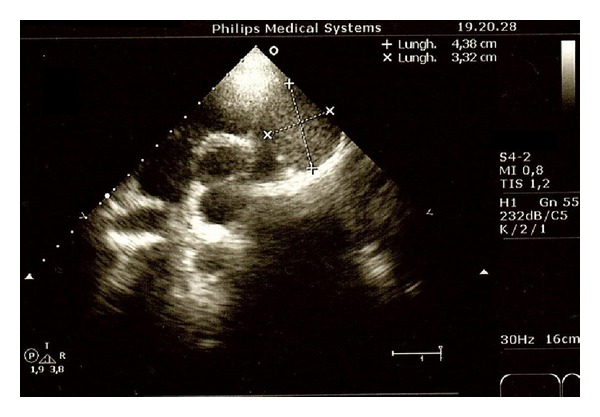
Transthoracic echocardiography with an ovalar iperecogenous mass of about 3.4 × 4.3 cm near the left side of the pulmonary trunk.
